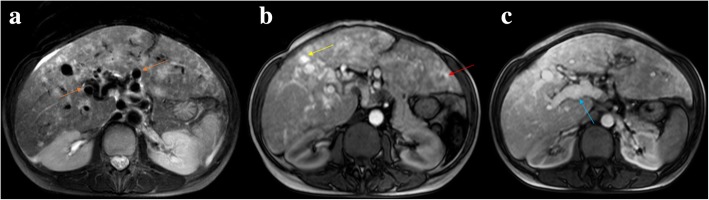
Hereditary hemorrhagic telangiectasia. a Axial fat suppressed T2-weighted MR image shows increased caliber of the hepatic artery and its branches (orange arrows). Note also the enlarged liver with heterogeneous texture. b, c Axial gadolinium-enhanced fat suppressed T1-weighted MR images in the arterial phase show intrahepatic telangiectasias, appearing as rounded small hyperenhancing lesions (b yellow arrow). An early contrast filling of portal venous branches is also seen, both peripheral (b red arrow) and central branches (c blue arrow), in addition to an increased PV caliber
